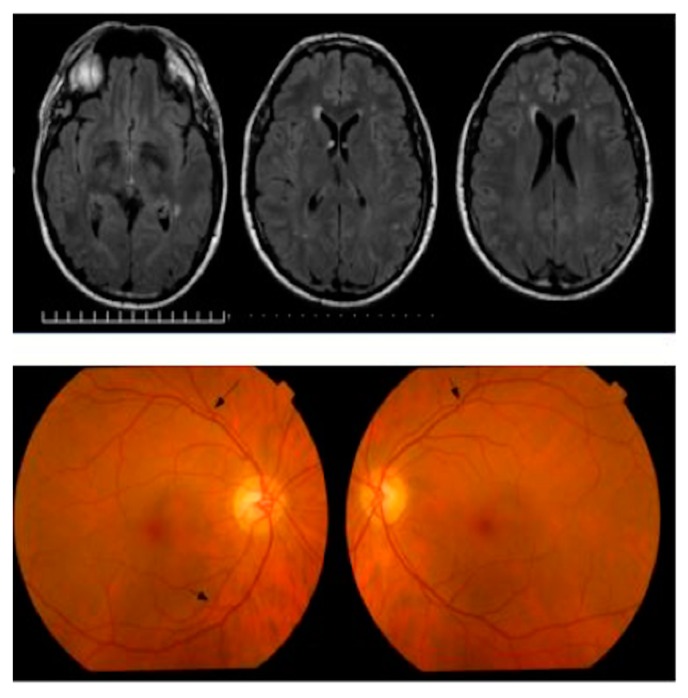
Magnetic resonance images (MRI) and fundus images from a supposedly healthy subject (male, 51 years old) with discrete focal lesions in the brain without visible or detectable ocular manifestations. TOP: MRI images showing mild to moderate white matter (WM) disease in the MRI image. The images show discrete focal lesions in anterior and posterior WM, focal confluence in posterior WM, and periventricular caps. BOTTOM: Fundus images annotated (see black arrows) showing arterio-venous crossings. The arterio-venous crossings are frequent in the average healthy population. (Image Courtesy of Valia Rodriguez at Aston University, personal communication).
